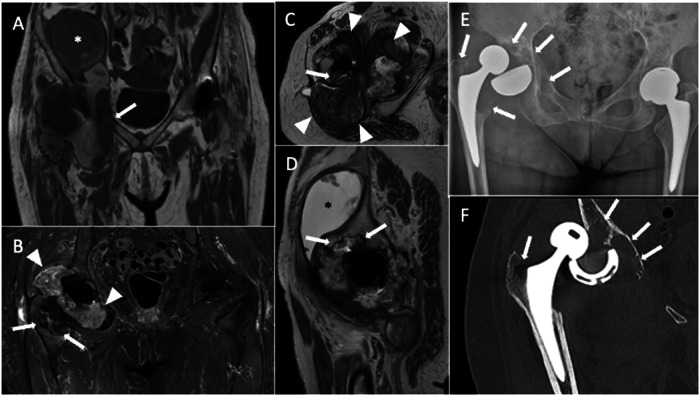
A 90-year-old female with bilateral THA and metallosis on the right. Coronal T1-weighted (A), coronal STIR (B), axial T2-weighted (C), and sagittal T2-weighted (D) images show T2-hypointense periprosthetic lobular mass with demarcated low-signal rim (arrowheads) and extensive bone resorption in acetabular, femoral, and superior ileo-pubic ramus (arrows). Also, note the remarkable distension of iliopsoas bursa (*). Five days later, before revision surgery, spontaneous THA dislocation occurred, as shown by plain radiography (E) and CT (F), which also highlight periprosthetic bone resorption (arrows)
